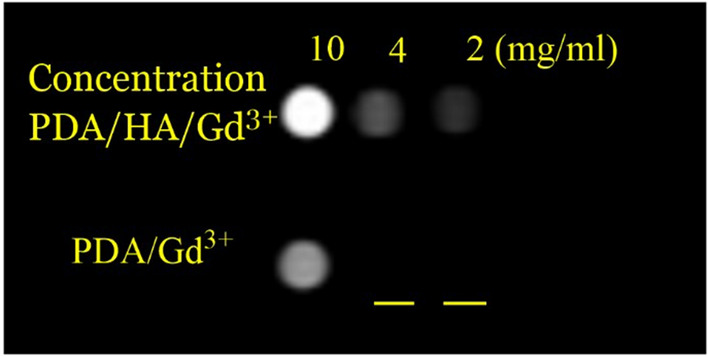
In vitro targeting MRI test. In vitro T1 MRI images of MDA-MB-231 cells treated with the PDA/HA/Gd^3+^ and PDA/Gd^3+^ CAs.
